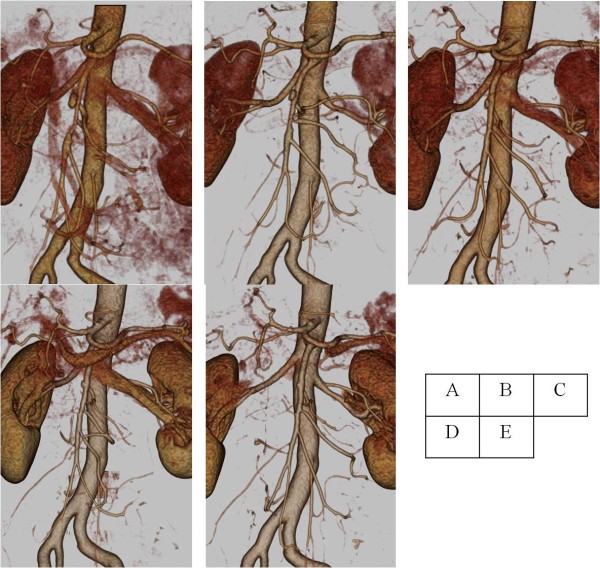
Change in the superior mesenteric artery ((A), first examination; (B-E), one month, two months, three months and one year later, respectively). Three-dimensional computed tomography showed a gradual re-expansion of the true lumen and improvements in the irregular arterial wall.
